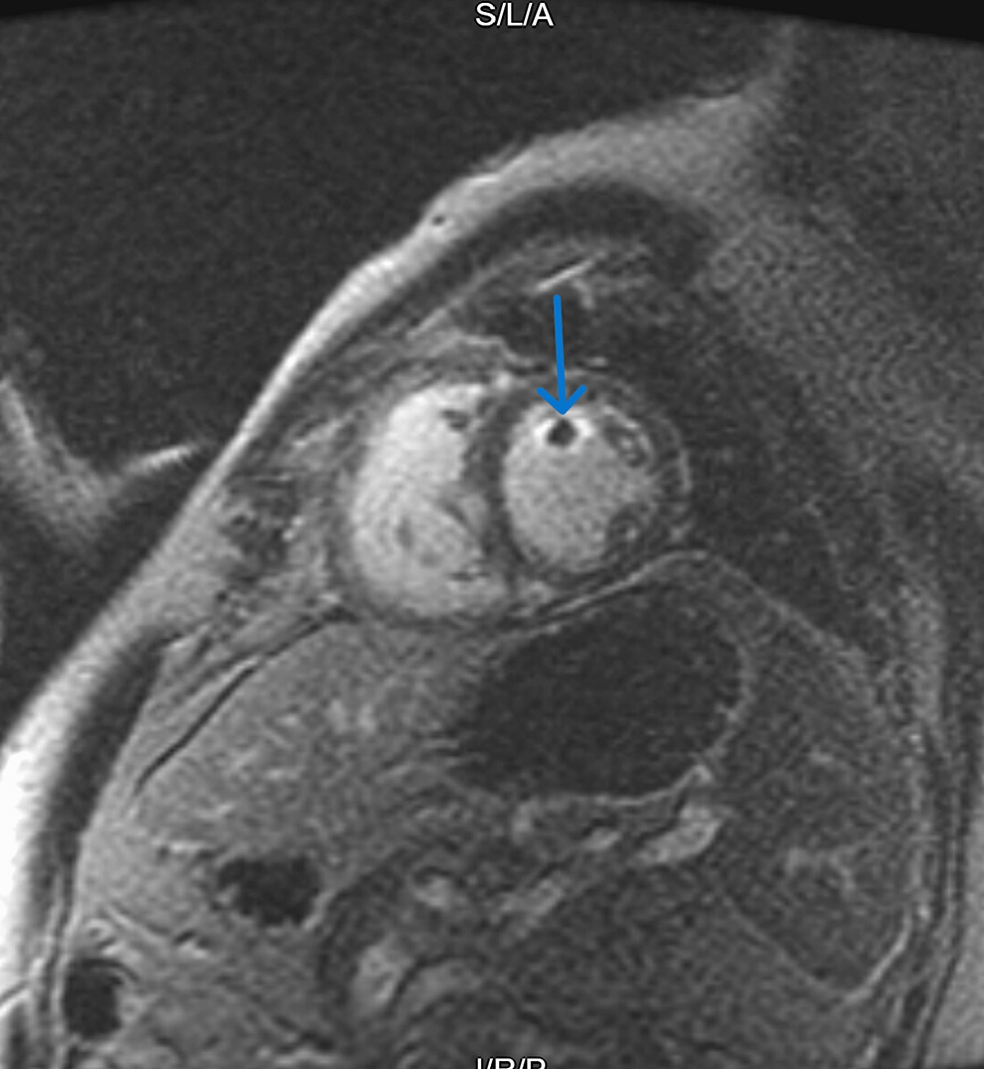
Cardiac MRI showing post-contrast peripheral enhancement of the left ventricular mass.
